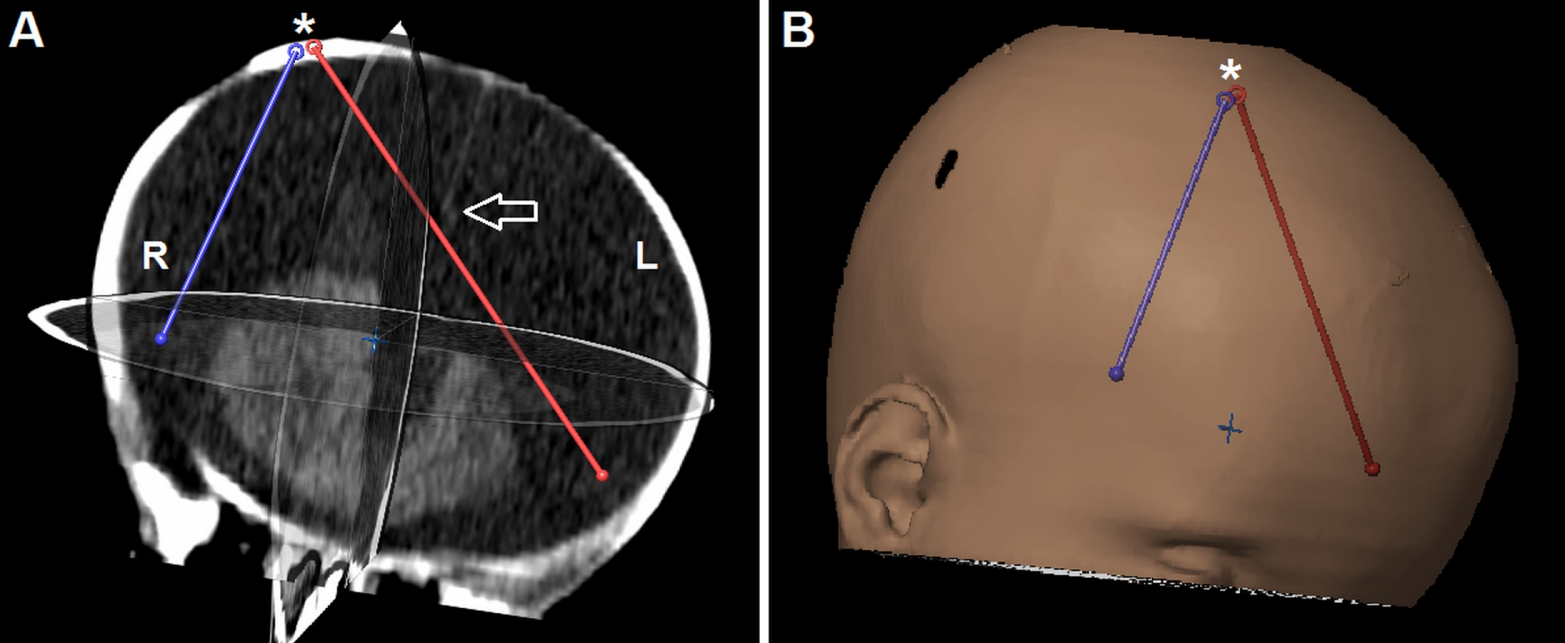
Preoperative virtual planning Preoperative virtual planning based on cranial computed tomography (A), showing the entry point (*) and endoscopic trajectories (red and blue lines). Of note, the red trajectory allows safe passage of the endocope below the falx (arrow) to reach the contralateral subdural space. (B) A three dimensional reconstruction demonstrates the right frontal coronal entry point.
